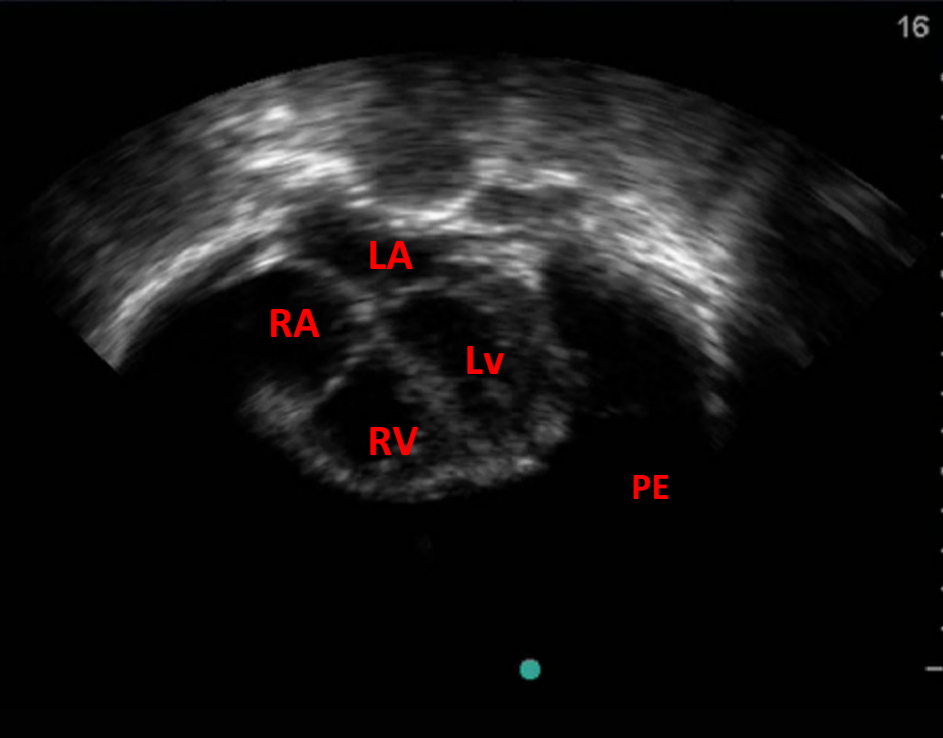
Apical Four Chamber view showing large pericardial effusion PE, RA, Right atrium; RV, Right ventricle; LA, Left Atrium; LV, Left ventricle.
